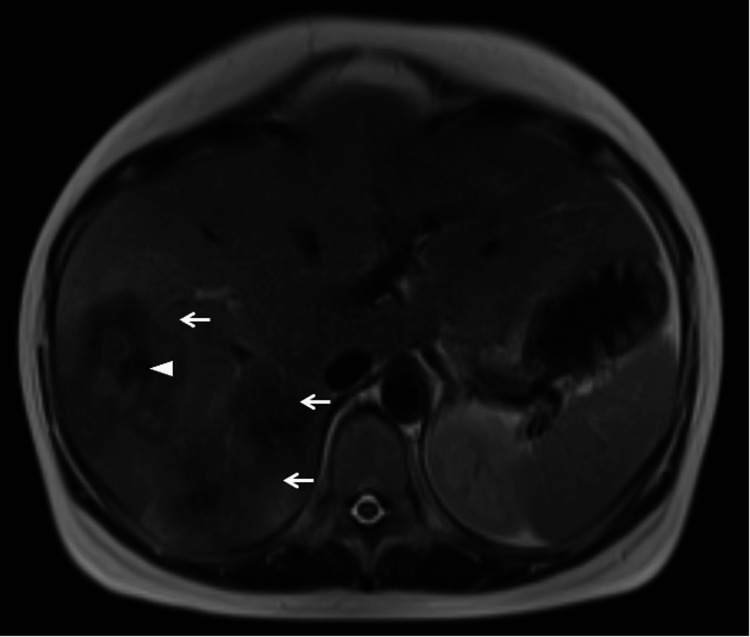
Non-contrast magnetic resonance imaging (MRI; T2-weighted) revealed multiple hepatic lesions (←) with internal necrosis (◁)
